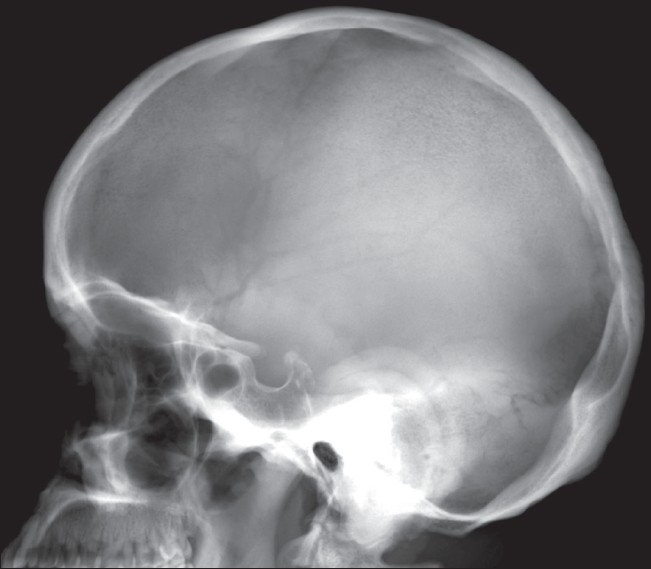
Lateral radiograph of the skull shows hyperostosis of the calvaria and skull base bones with a normal sella and sinuses
